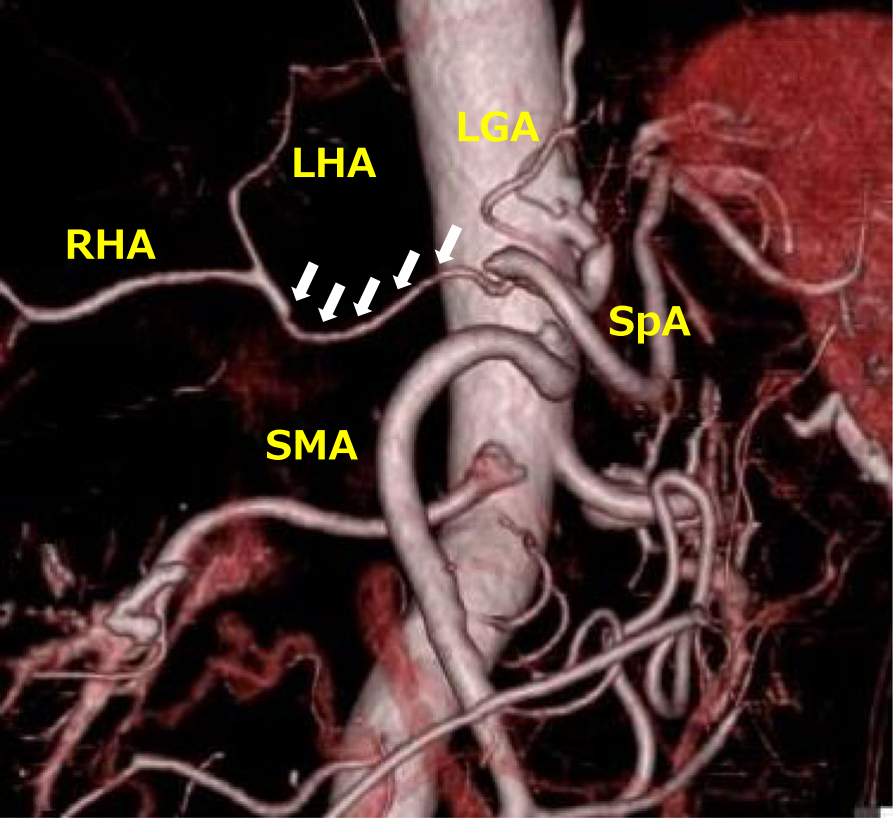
Follow-up CT angiography at 3 months after surgery demonstrated sufficient hepatic flow through the anastomotic site between the CHA and PHA (white arrow)
